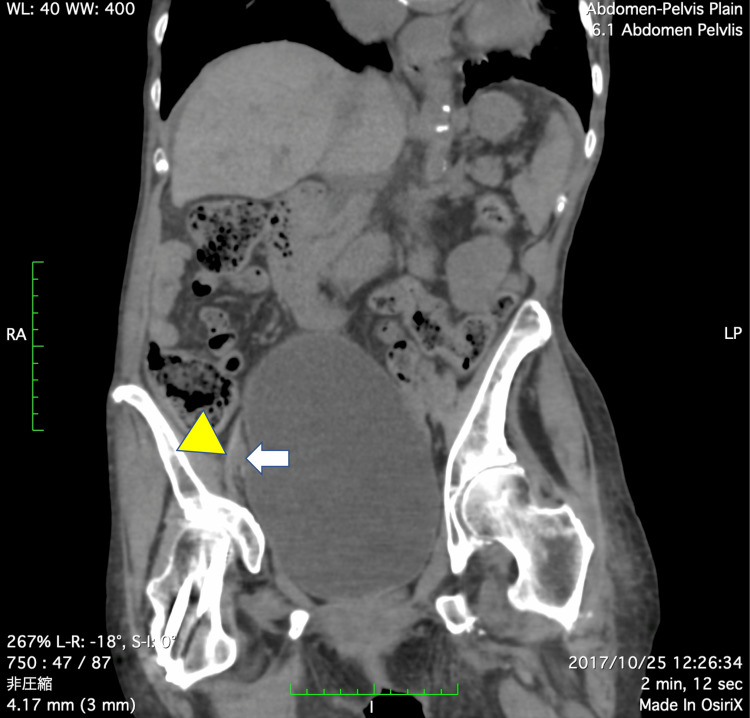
Computed tomography scan. Abdominal and pelvic computed tomography scans show the markedly distended bladder and right external iliac artery (white arrow) compressing the right external iliac vein (yellow triangle).
